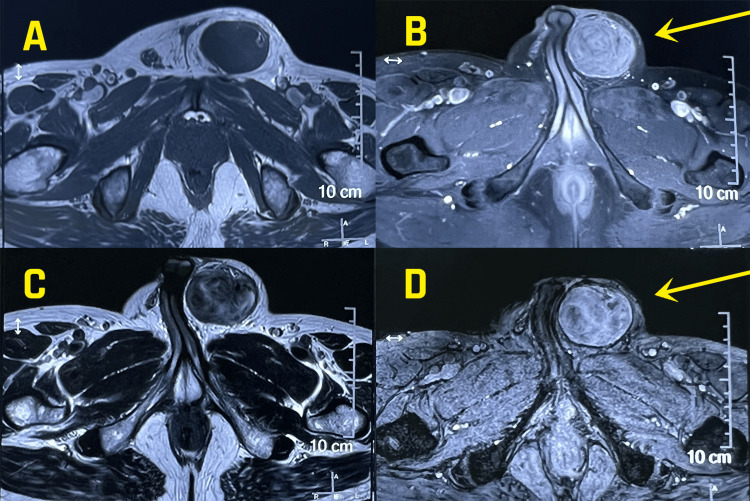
MRI axial view (A) and (B) showing T1W pre and post contrast images showing left inguinoscrotal mass with diffuse enhancement(arrow); (C) T2W image showing heterogenous signal in the lesion; (D) GRE image showing areas of blooming (arrow). GRE: gradient echo
